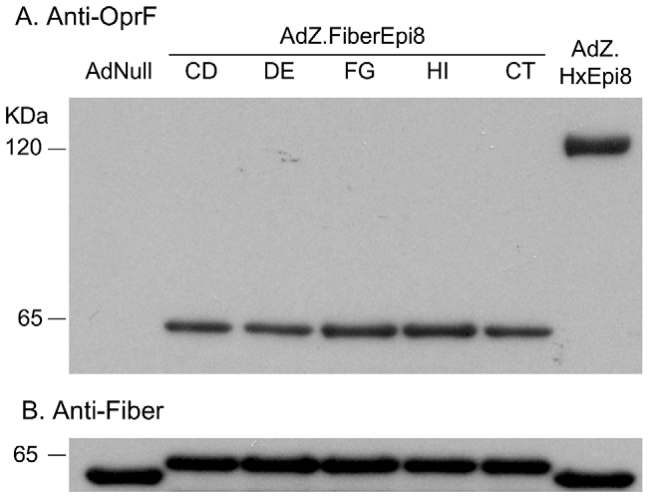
P. aeruginosa* Epi8 epitope in various Ad vector fiber sites. The fiber-modified Ad vectors AdZ.F(CD)Epi8, AdZ.F(DE)Epi8, AdZ.F(FG)Epi8, AdZ.F(HI)Epi8 and AdZ.F(CT)Epi8, the hexon-modified AdZ.HxEpi8 or AdNull as control (all 10^10^ particles) were separated on 4–12% polyacrylamide gradient SDS-PAGE, transferred to a polyvinylidine difluoride membrane and evaluated by Western blot analysis using A. anti-OprF serum; or B. anti-Ad fiber antibody.
